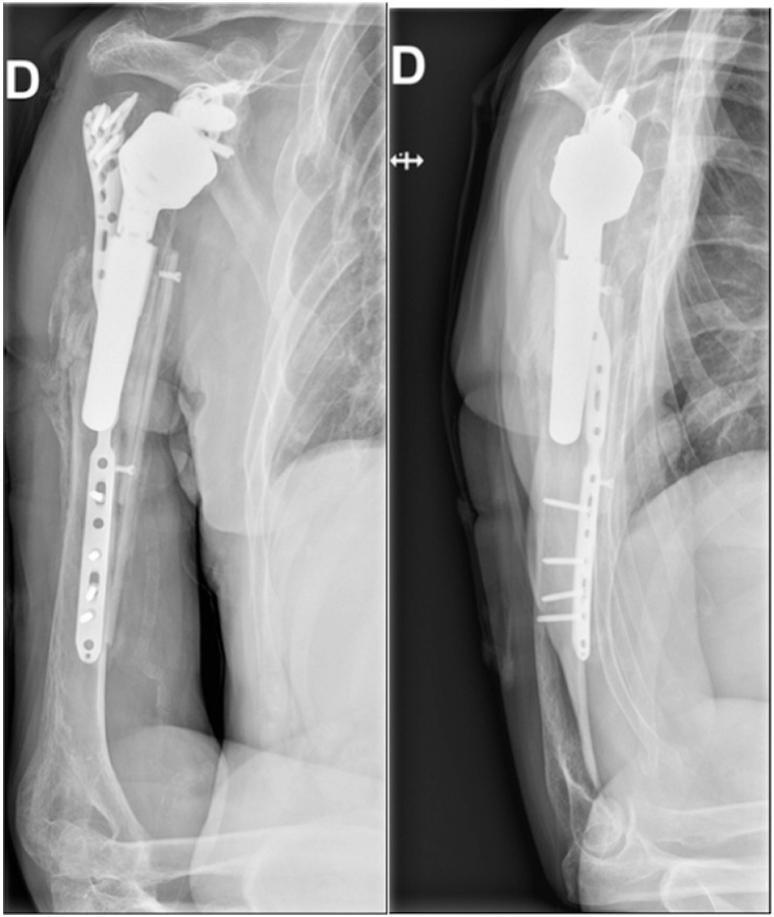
Six weeks postoperative X-rays.
